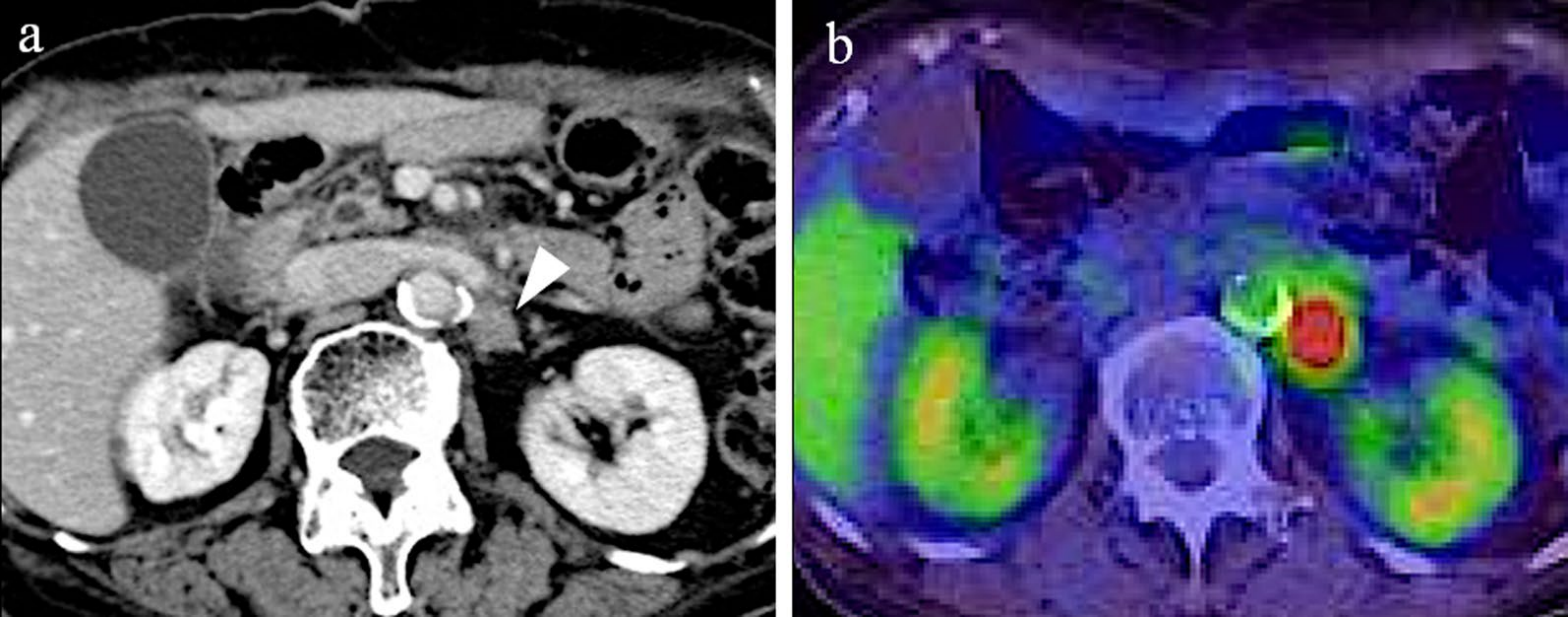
a Contrast-enhanced CT and b fluorodeoxyglucose-positron emission tomography/CT at recurrence of cancer appeared in No. 16b1. The size was 14.6 mm
